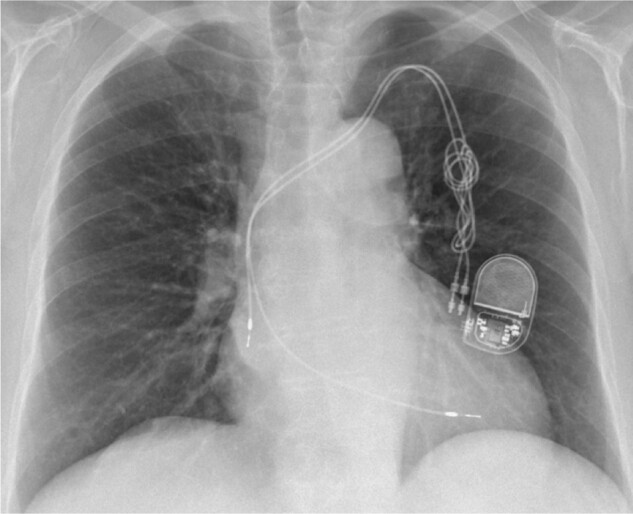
Case report 2. Chest X-ray—7 months later.
